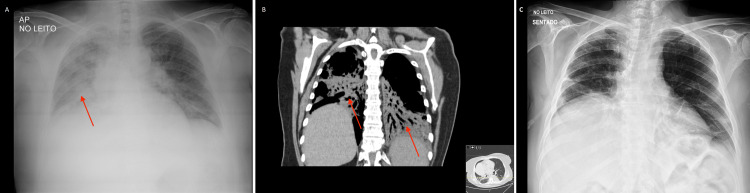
Imaging evolution during hospitalization. (A) Chest X-ray before admission to the Intensive Care Unit (red arrow). (B) Coronal cut of chest CT showing bilateral pneumonia (two red arrows). (C) Chest X-ray after five-day treatment with azithromycin, showing resolving pneumonia.
